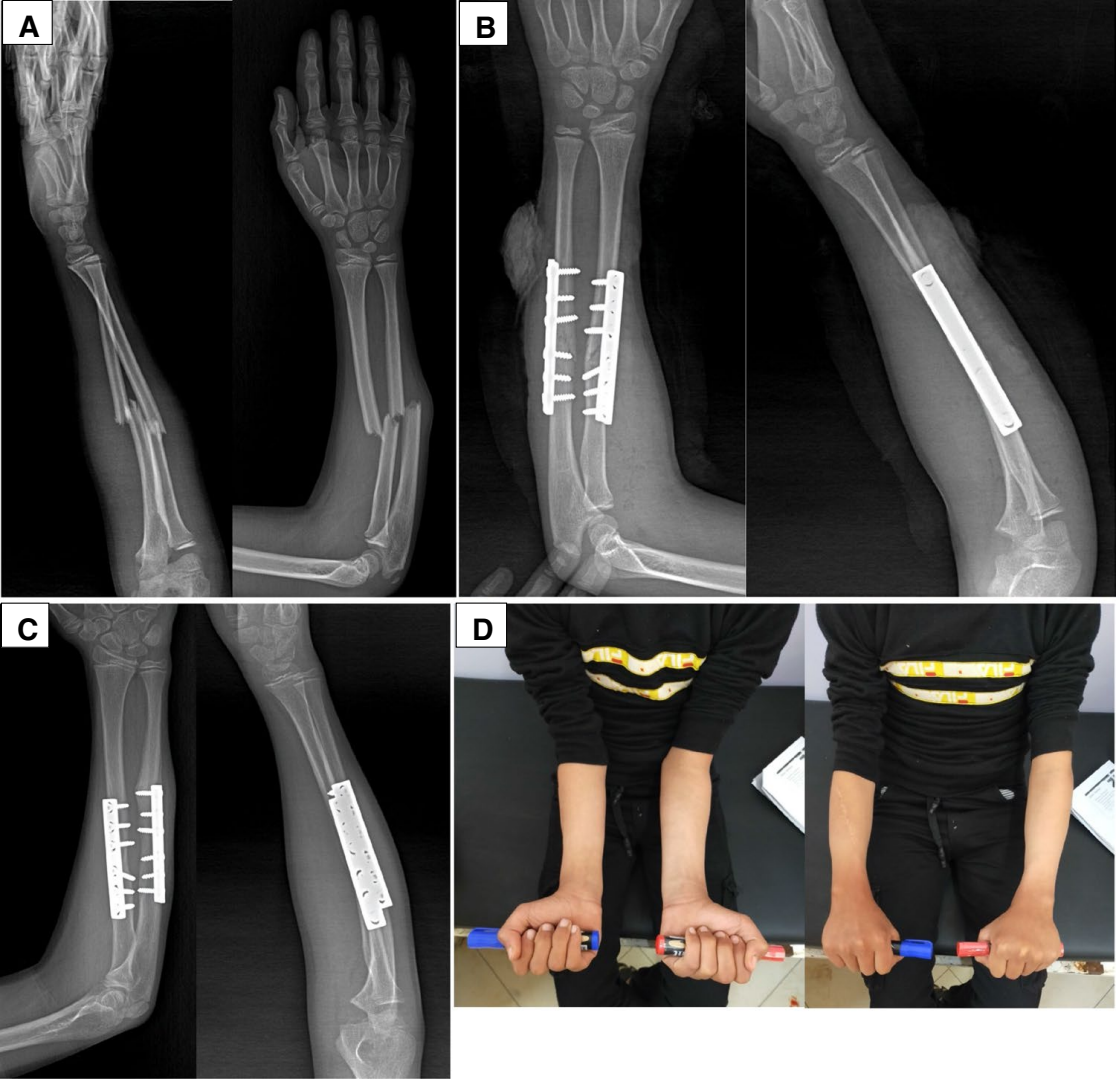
Fourteen-year-old male child diagnosed with right both-bone unstable forearm fracture following falling on outstretched hands. A—Pre-operative X-rays (AP & lateral) showing mid shaft forearm fracture with shortening. B—Post-operative X-rays (AP & lateral) showing both-bone fixation. C—X-rays taken at 6 weeks post-operatively confirm radiological union. D—Clinical photograph showing comparable forearm range of motion
